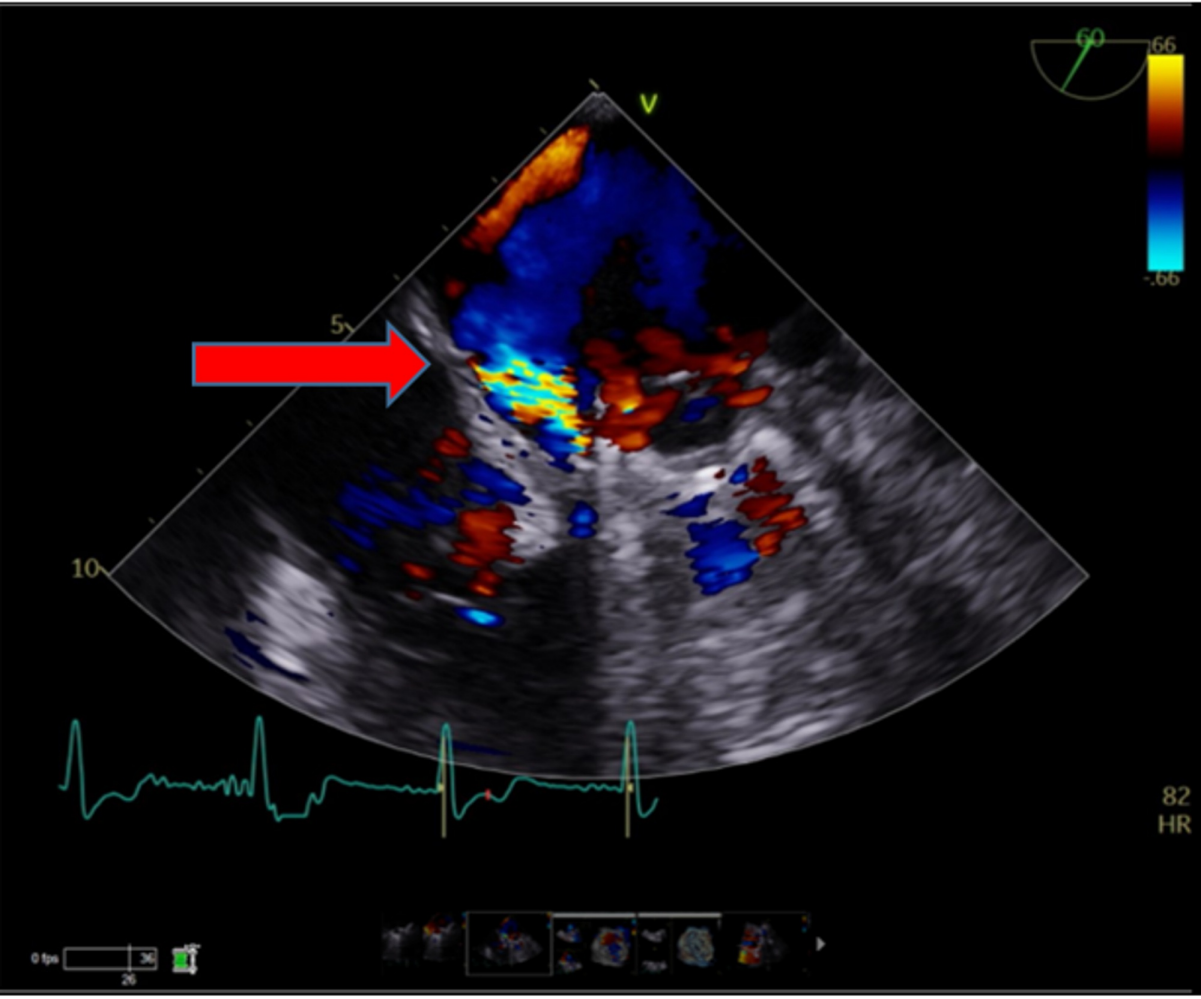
Mitral valve prosthesis with paravalvular regurgitant jet (red arrow) on trans-esophageal echocardiogram
